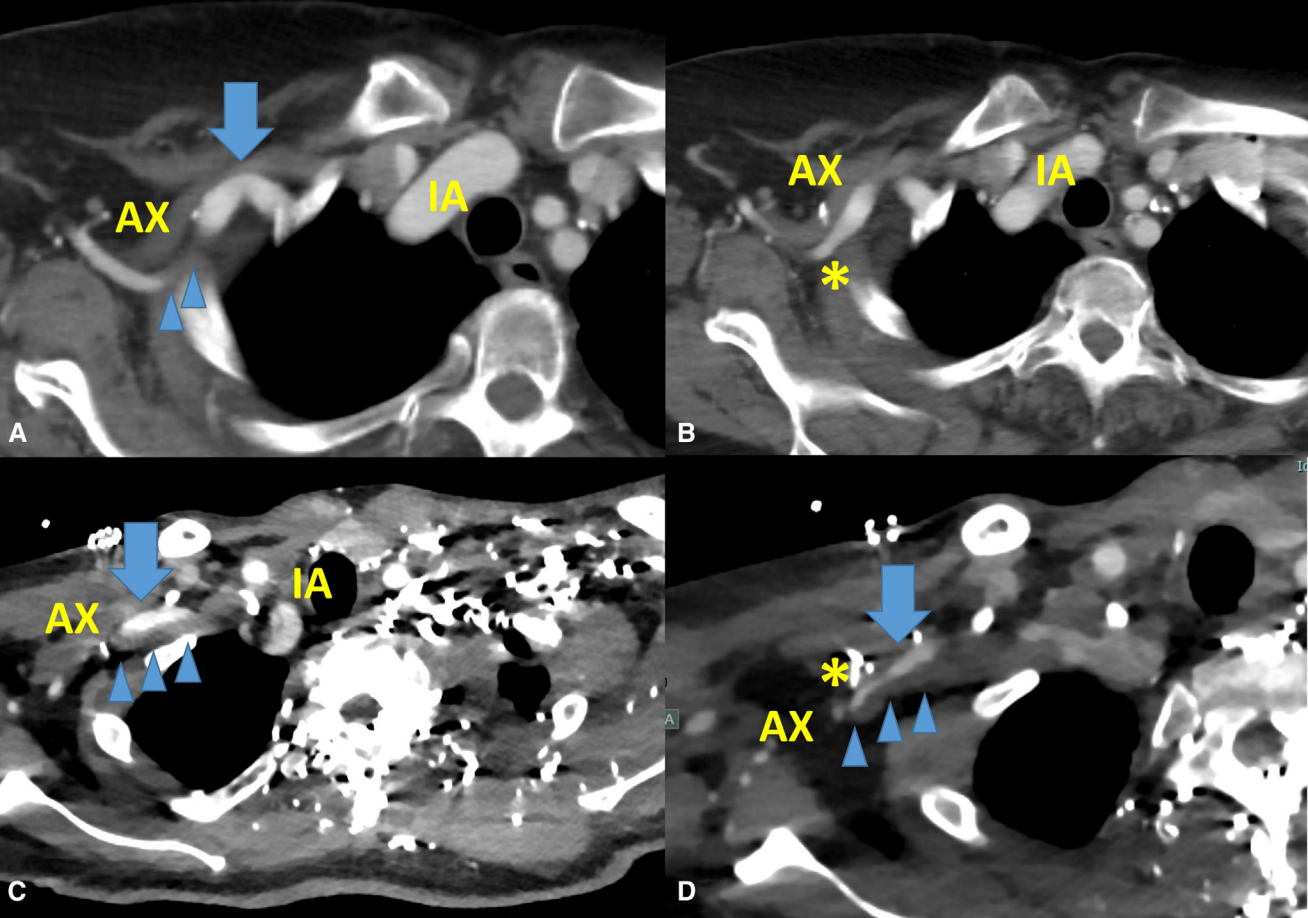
Failure cases of redo axillary artery (AX) cannulation. A and B, An 83-year-old woman with chronic dissection. A torturous AX to the subclavian artery was noted. The asterisk showed the old cannulation site. Redo direct axillary cannulation with an angled cannula was unsuccessful. The presumable cause was the acute turn of a proximal AX (*arrow*), which does not allow insertion of an arterial cannula. C, and D, A 45-year-old man with prior history of type A dissection repair. Preoperative computed tomography angiography showed a dissected AX and right subclavian artery with a thrombosed false lumen (*arrowheads*). A true lumen (*arrow*) of the right AX was 4.5 mm in diameter. Redo direct AX cannulation was performed but arterial back flow was insufficient. *IA*, Innominate artery. ∗The old cannulation site.
